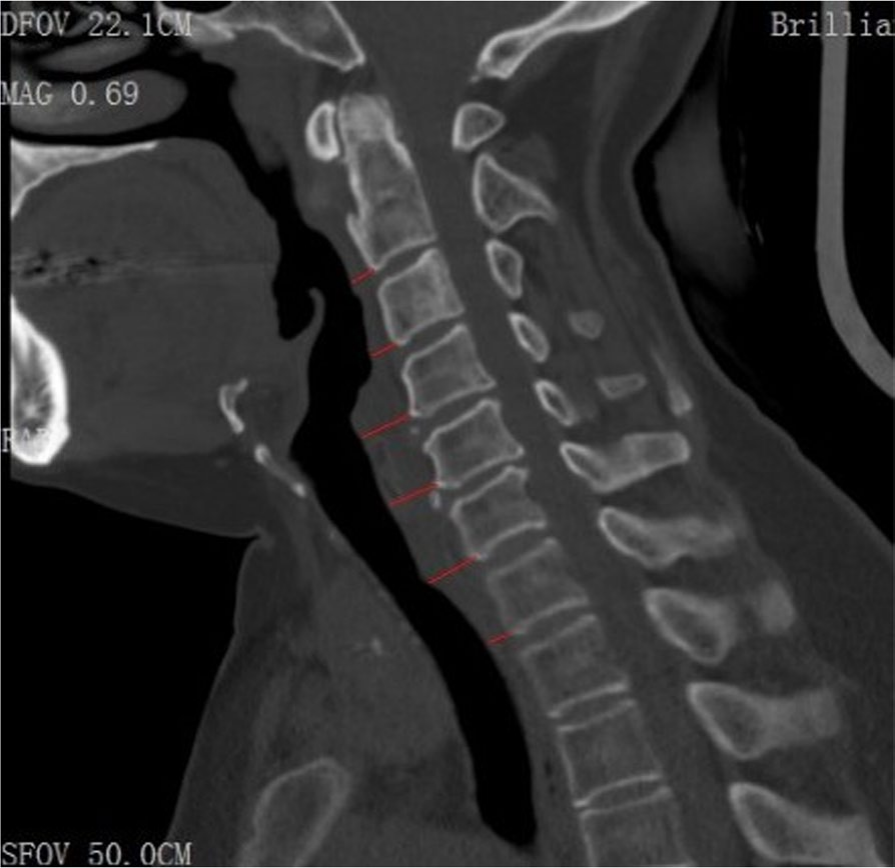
The PSTS was measured between the antero-inferior corner of each vertebral body and the air shadow of the airway through CT mid-sagittal slice images
